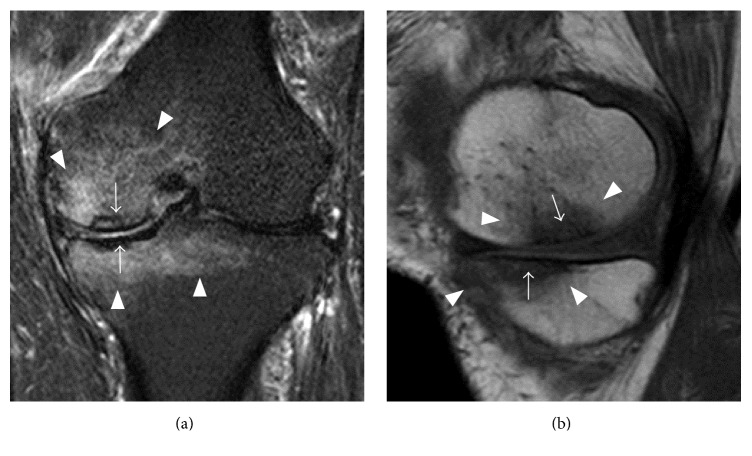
Initial MRI evaluation of our patient. (a) Coronal STIR and (b) sagittal T1 weighted images showing focal SPONK lesions at both the MFC and the MTP (arrow), surrounded by diffuse bone edema (arrowhead). MRI also showed a horizontal tear in the medial meniscus at the posterior horn.
